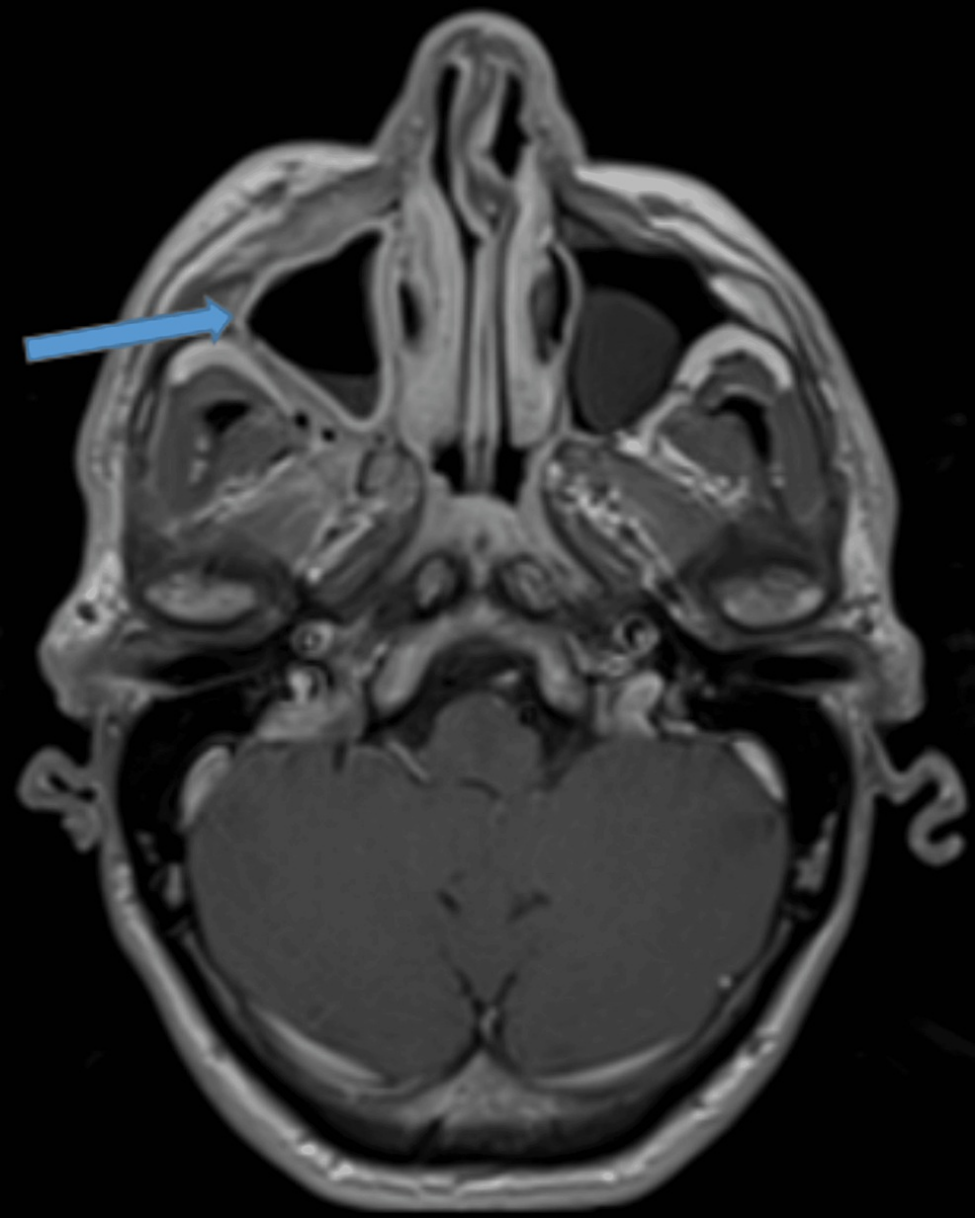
T1-weighted post-contrast MRI – axial image Enhancing inflammatory change within the soft tissues of the right maxillary wall (blue arrow).
